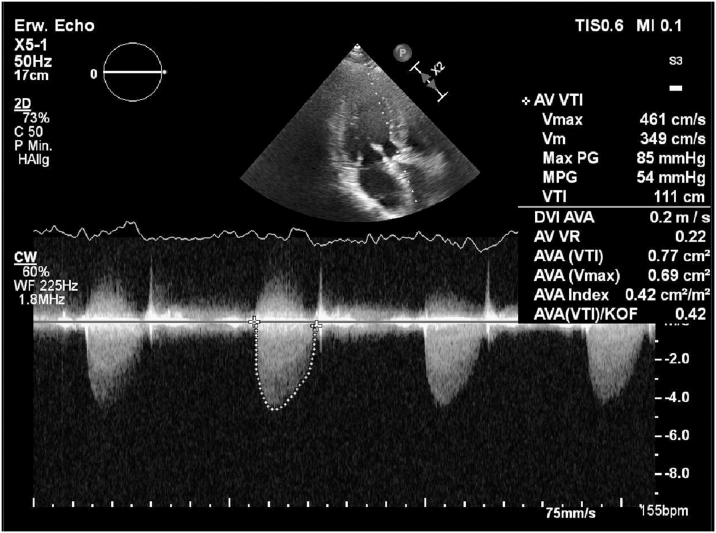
Measurement of the Severely Stenotic Neo-Aortic Valve by Transthoracic Echocardiography
